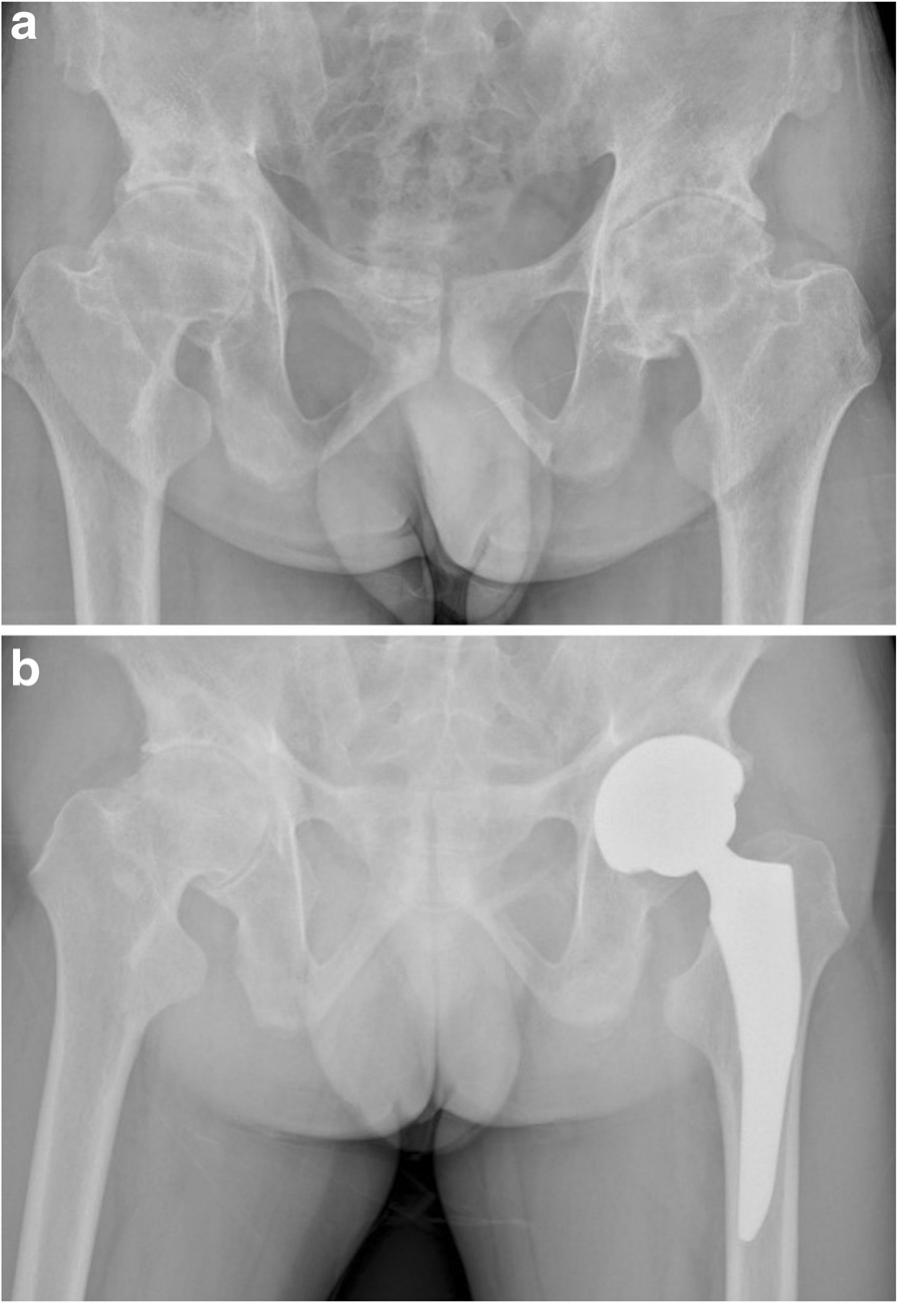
a A preoperative radiograph of the hip of a 73-year-old man who had bilateral hip osteoarthritis. b A radiograph at 38 months after implantation of short cementless femoral stem in the left hip showed solid fixation in a satisfactory position
